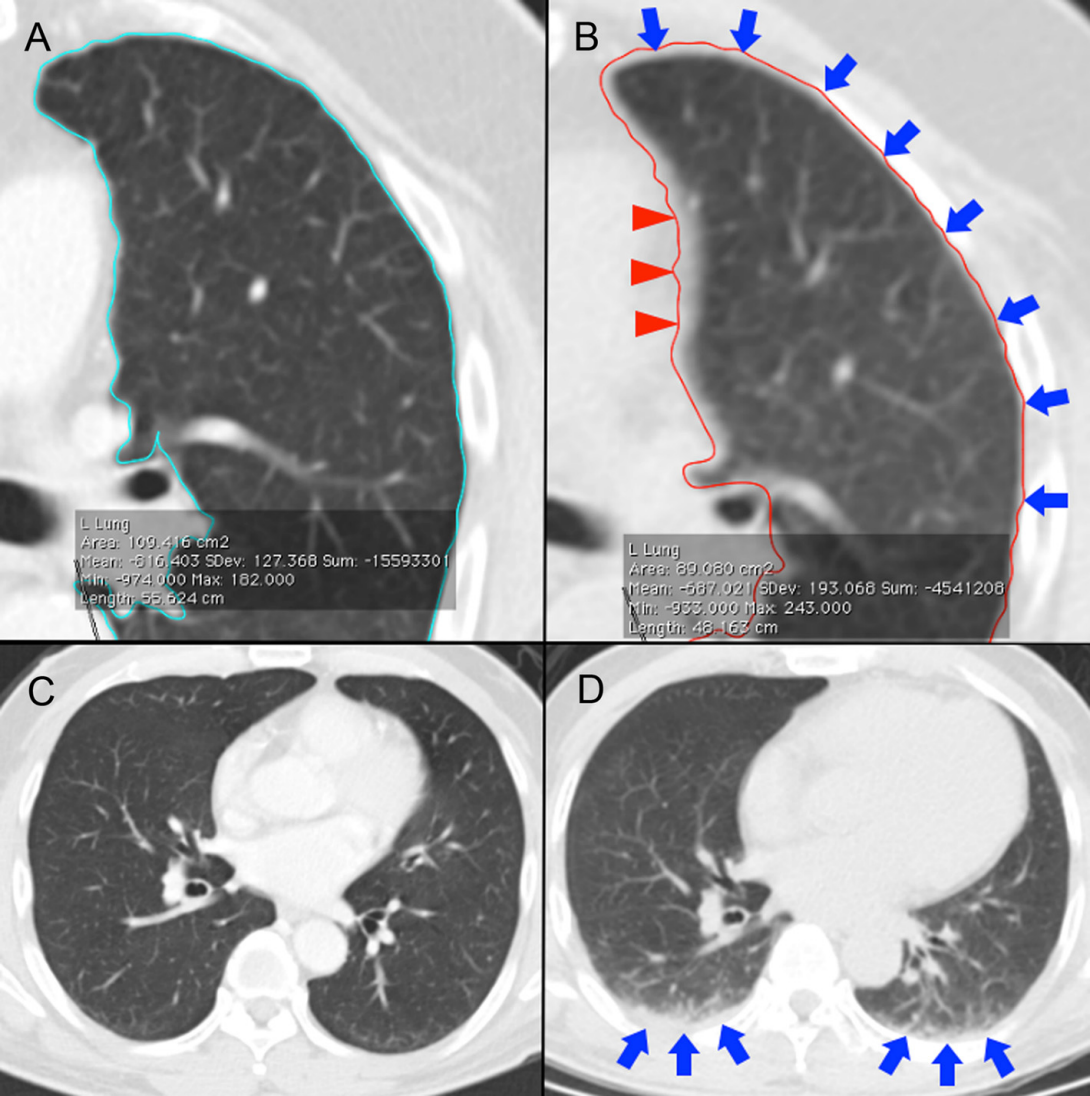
Technical considerations in region-of-interest (ROI) delineation. On diagnostic CT, auto-segmented ROIs should closely match lung parenchyma margins (A). Our SPECT/CT ROI technique requires the inclusion of a very thin sliver of pleura within its margins (blue arrows), but this has a tendency to over-include the left mediastinal border (red triangles) (B). Due to differences in CT scan technique, diagnostic CT (C) and SPECT/CT (D) of the same patient performed a week apart show dependent atelectasis on SPECT/CT (D, blue arrows) but not on diagnostic CT (C).
